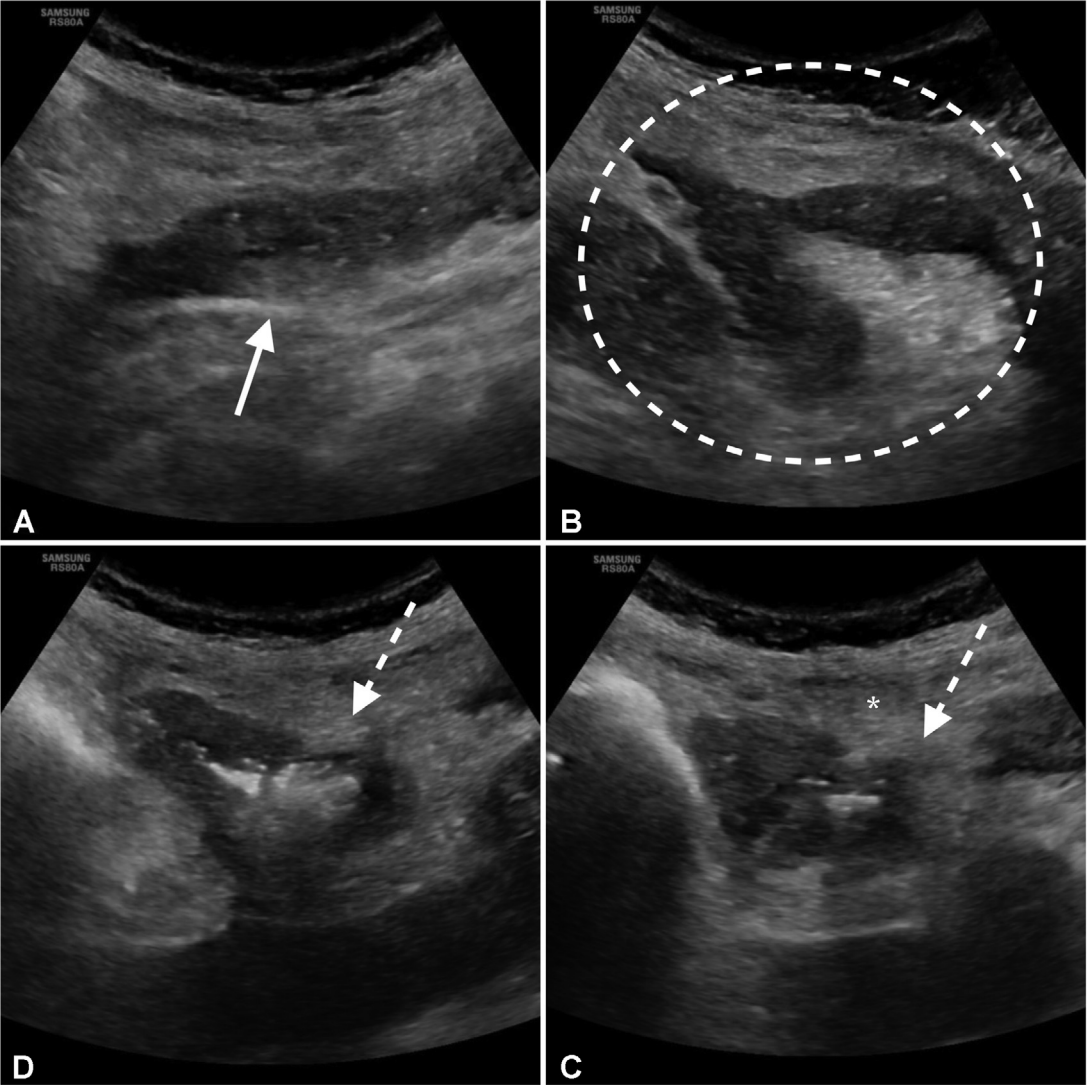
(A-D) Ultrasound examination with convex probe. At the left lumbar region, a small bowel loop with eccentric thickening was appreciated with ill-defined margin on the mesenteric side (with arrow) (A) Adjacent small bowel loops were collapsed and angled (dashed circle) (B) An outpouching lesion with thickened walls and mixed content was detected in connection with a bowel pool (dashed arrow) (longitudinal C, axial D). The adjacent mesenteric fat was inhomogeneous, thick, and hyperechogenic (asterix).
